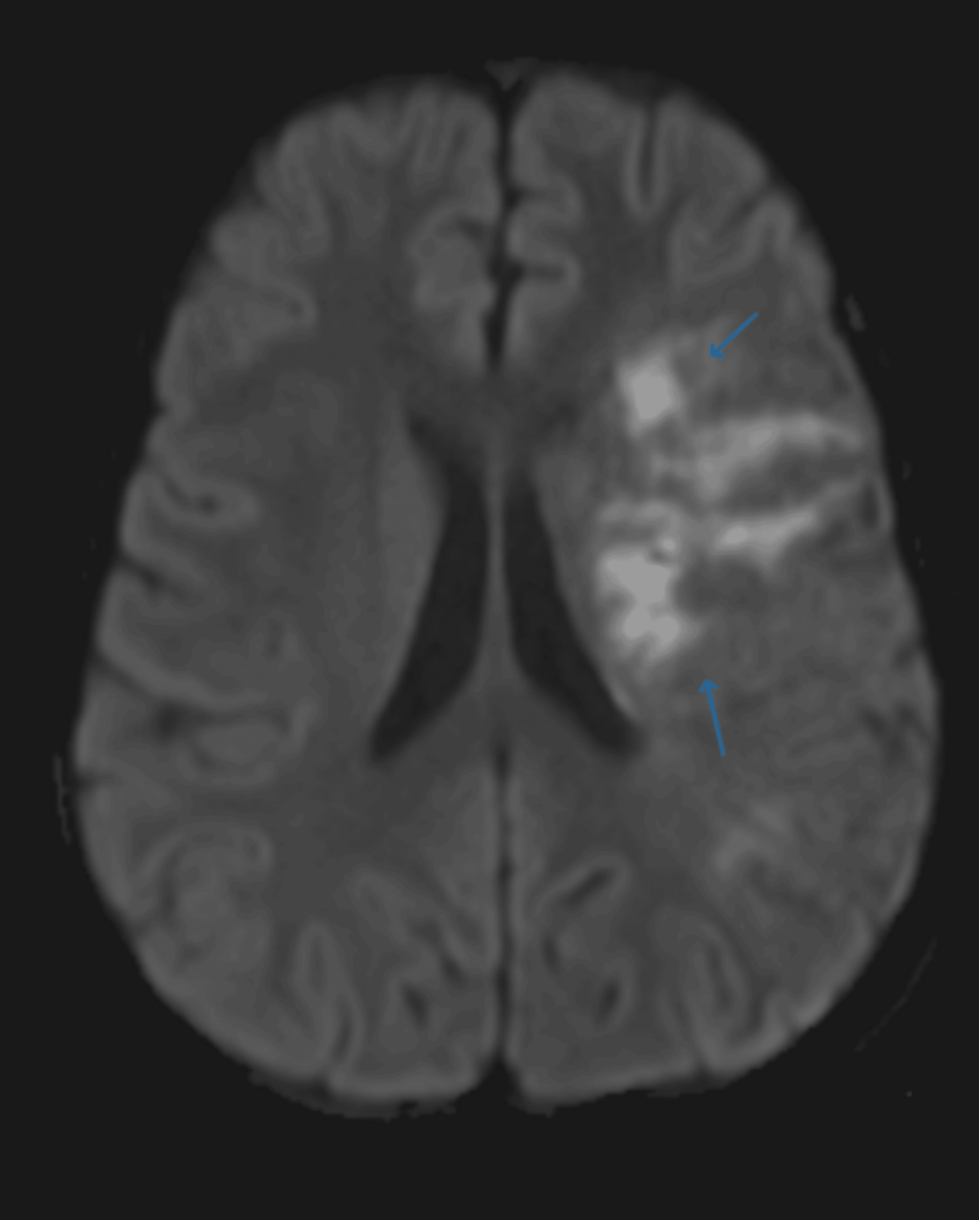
Diffusion-weighted imaging (DWI) showing restricted diffusion in the left frontoparietal region, confirming acute ischemic infarct.
